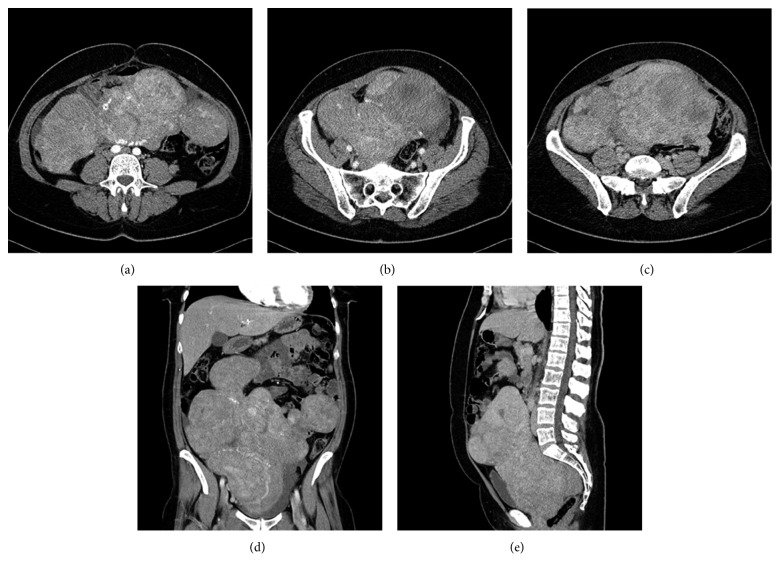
CT scan of the monstrous uterus myomatosus of a 47-year-old nulliparous patient.
